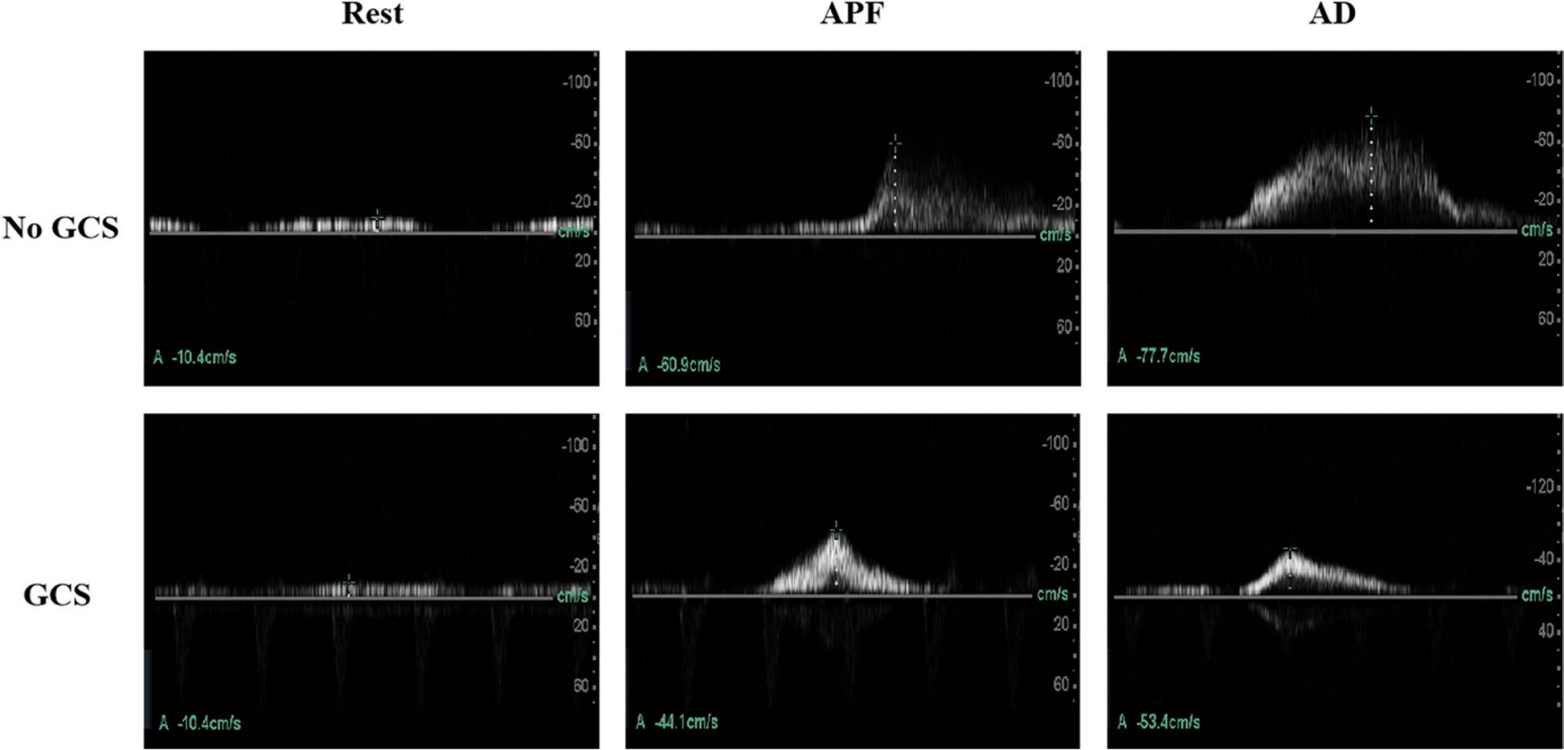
Ultrasonic Doppler images of femoral venous peak velocity during rest, ankle plantar flexion and ankle dorsiflexion, with and without compression
